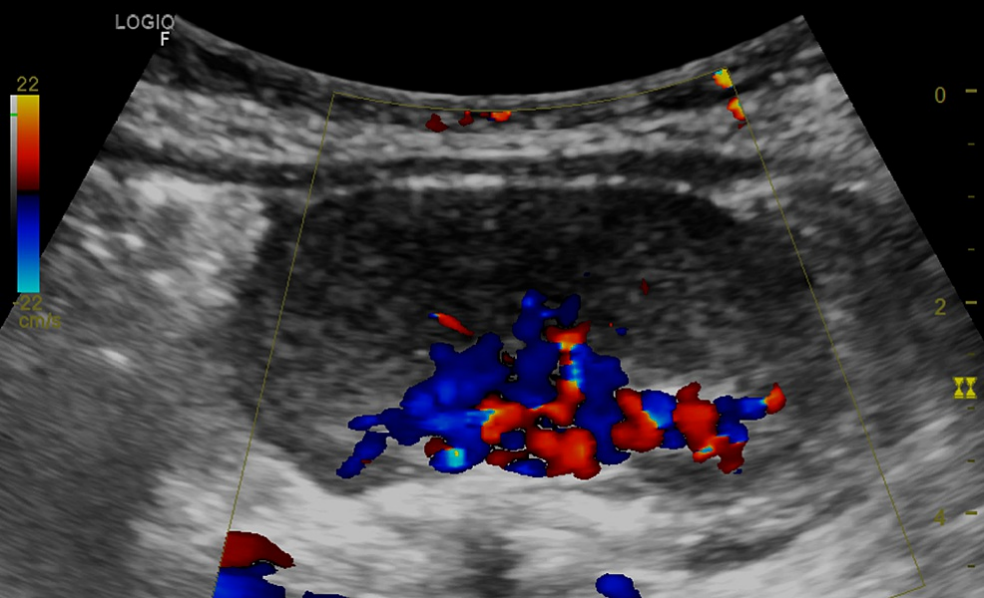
Abdominal ultrasound. A solid lesion in the left iliac fossa with heterogeneous echogenicity and significant arterial and venous vascularization in color Doppler mode.
